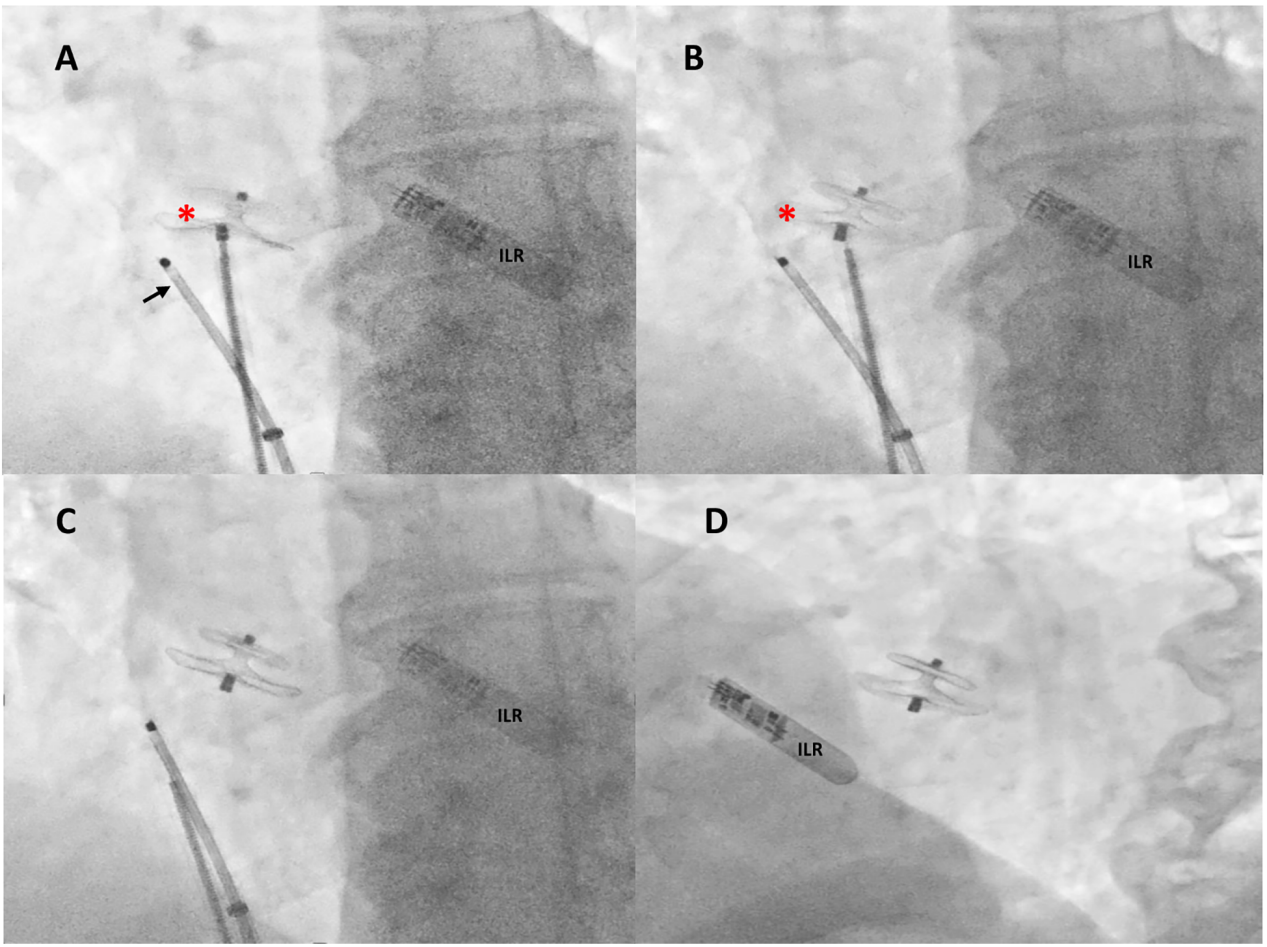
Intraprocedural fluoroscopic procedural steps. Under rotational intracardiac echocardiography (black arrow) and using a dedicated 9-F-long sheath, a 18/24 mm MemoPart PFO occluder (red asterisk) connected by a microscrew to a delivery cable was positioned across the PFO tunnel (A), the device was then disconnected by turning the delivery cable counterclockwise (unscrewing) (B), and the final correct position of the device was confirmed by fluoroscopy in anteroposterior (C) and left anterior oblique 30° view (D). ILR, loop recorder.
